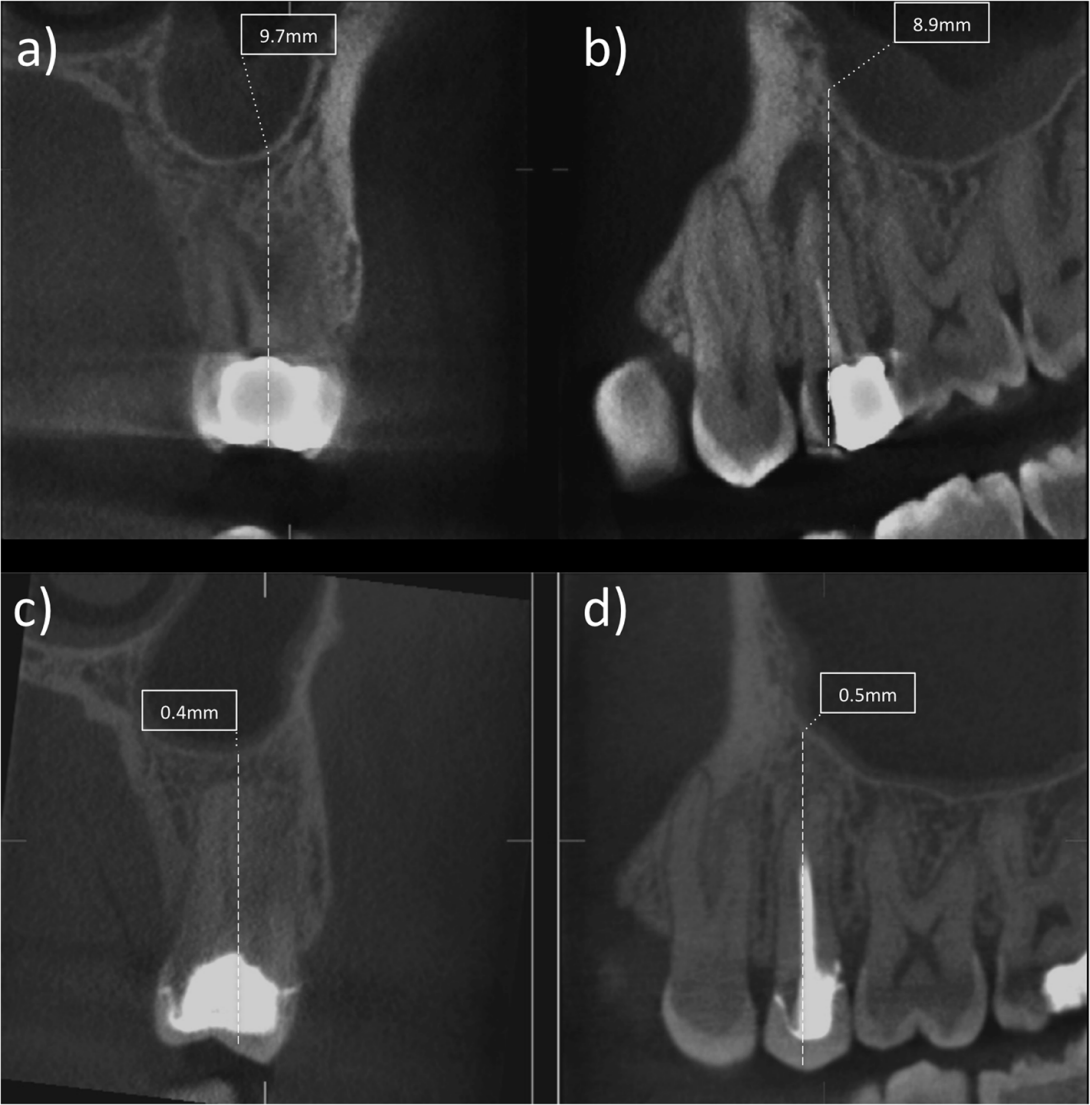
(a-d). Image showing the pre- and post treatment measurements on the coronal (a,c) and sagittal cone beam computed tomography slice (b,d) related to an upper left second molar area with the relative measurement of the Schneider membrane thickness in mm before root canal re-treatment (a,b) and after one year follow-up (c,d)
